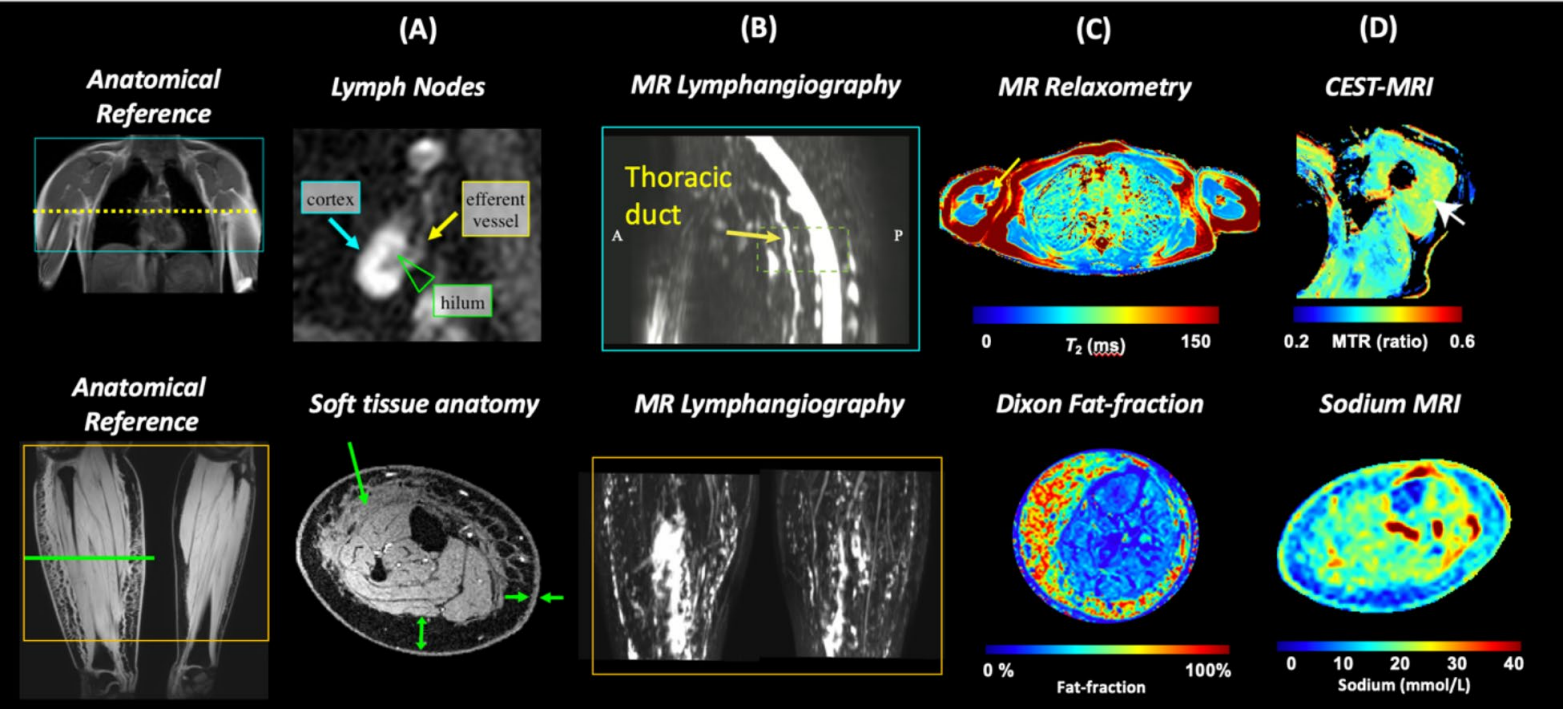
Noninvasive MRI methods for structural and physiologic lymphedema assessment. Noninvasive MRI methods provide structural and physiologic imaging measures for upper-extremity lymphedema (top row) and lower-extremity lymphedema (bottom row). A Optimized image contrast at 3T MRI provided lymph node anatomical imaging sensitive to the afferent and efferent vessel and lymph node substructures. Structural MRI of soft tissue anatomy demonstrates tissue remodeling in disease such as skin and adipose tissue thickening, and fibrosis deposition. B MR lymphangiography visualizes deep lymphatic vessel morphology, such as in the thoracic duct anterior to the spinal cord. Edema is also visualized as hyper-intense signal on non-tracer MR lymphangiography to localize dependent edema and response to manual therapies. C Tissue composition in lymphedema can be quantified with 3T MR relaxometry (e.g. T_2_ relaxation time map in upper-extremity unilateral lymphedema), and Dixon fat-fraction mapping. These measures indicate edematous and heterogeneous tissue in lymphedema, which responds to manual lymphatic drainage therapy. D Molecular imaging methods relevant for lymphedema include CEST-MRI of proteins (e.g. magnetization transfer ratio, MTR of amide proteins) and sodium MRI of endogenous tissue sodium content (mmol/L). Molecular imaging is being investigated for early biomarkers of edema formation and objective, localized measures of lymphatic disease severity. Together, MRI is a versatile modality to investigate lymphatic physiology and disease with high potential for clinical translation.
